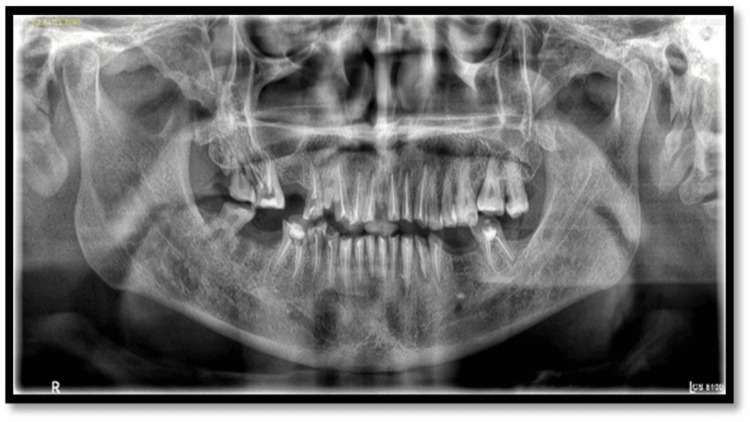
Post-endodontic panoramic radiograph
